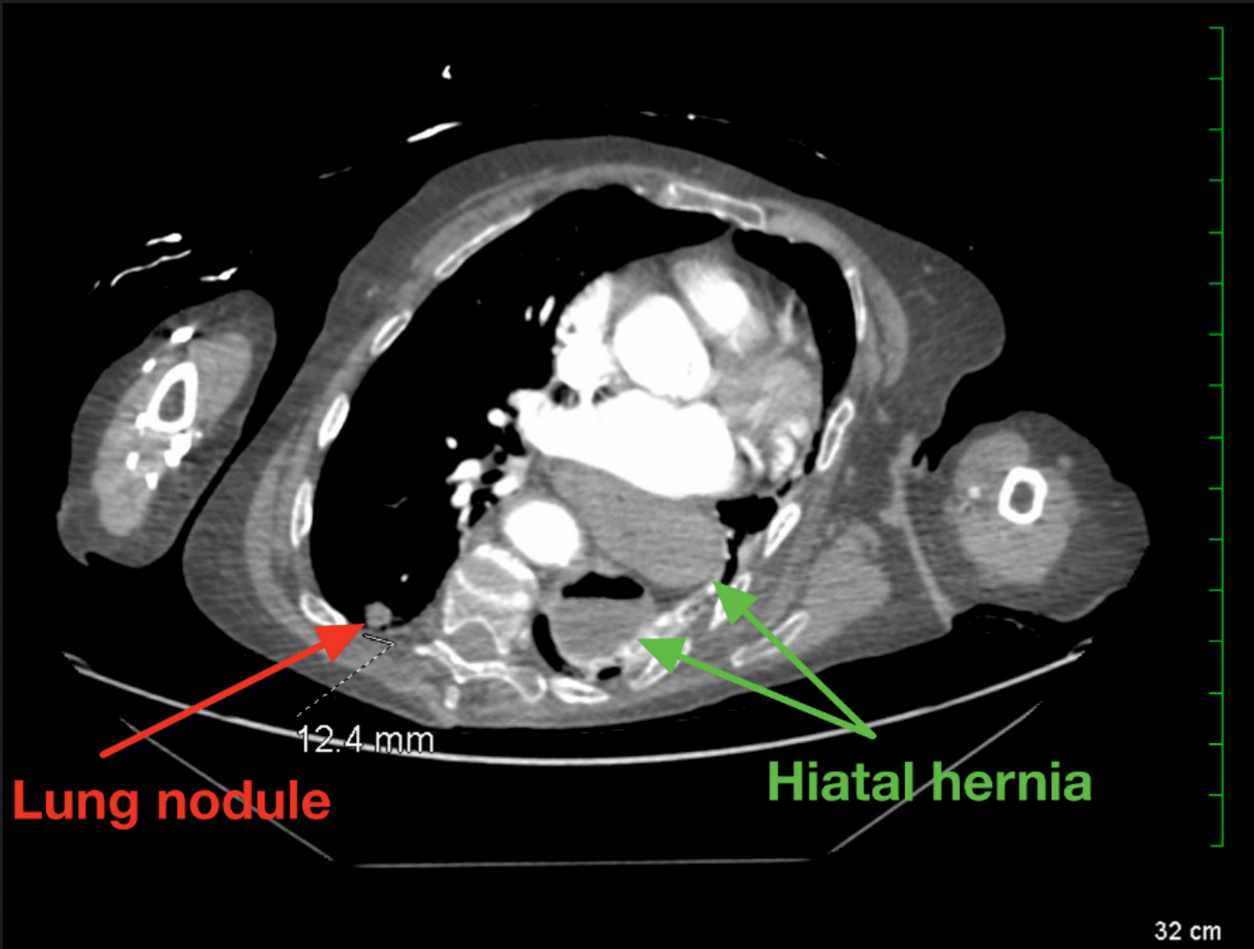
CT angiography of the chest showing a right lung nodule (red arrow) and a hiatal hernia (green arrows). CT: Computed tomography
